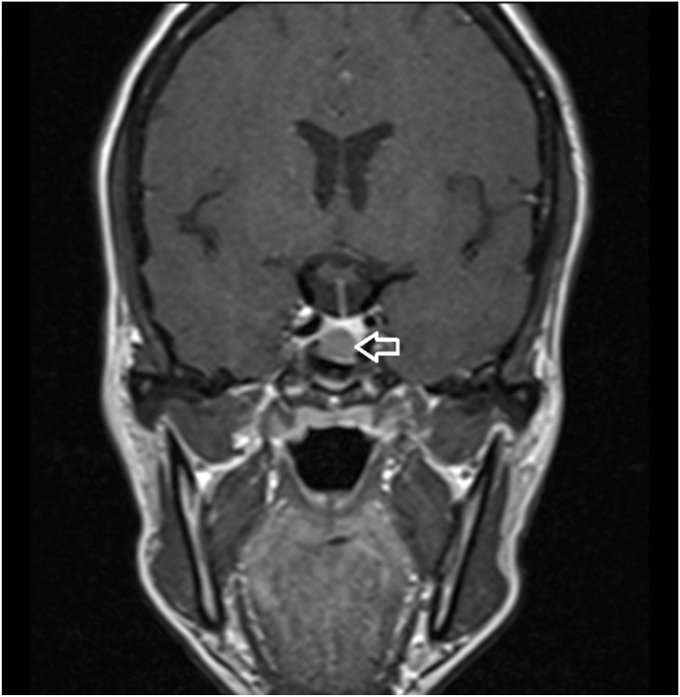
MRI of the sella turcica: Coronal T1-weighted image; the arrow points to the lesion in the adenohypophysis measuring 8.9 × 8.8 mm; slight deviation of the infundibulum; no invasion detected. *MRI* = magnetic resonance imaging.
